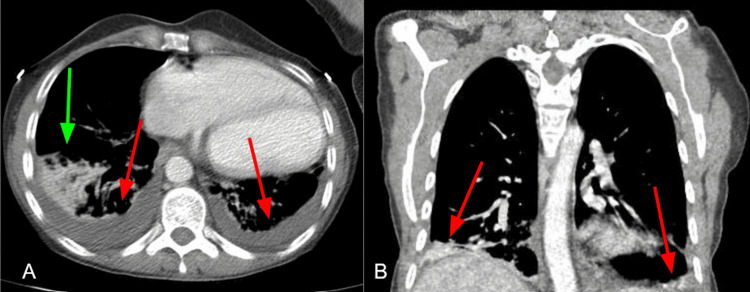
Chest window of CT scan obtained on admission Transverse (A) and coronal (B) view showing small bilateral pleural effusions (red arrows) with compressive atelectasis (green arrow) in A.
